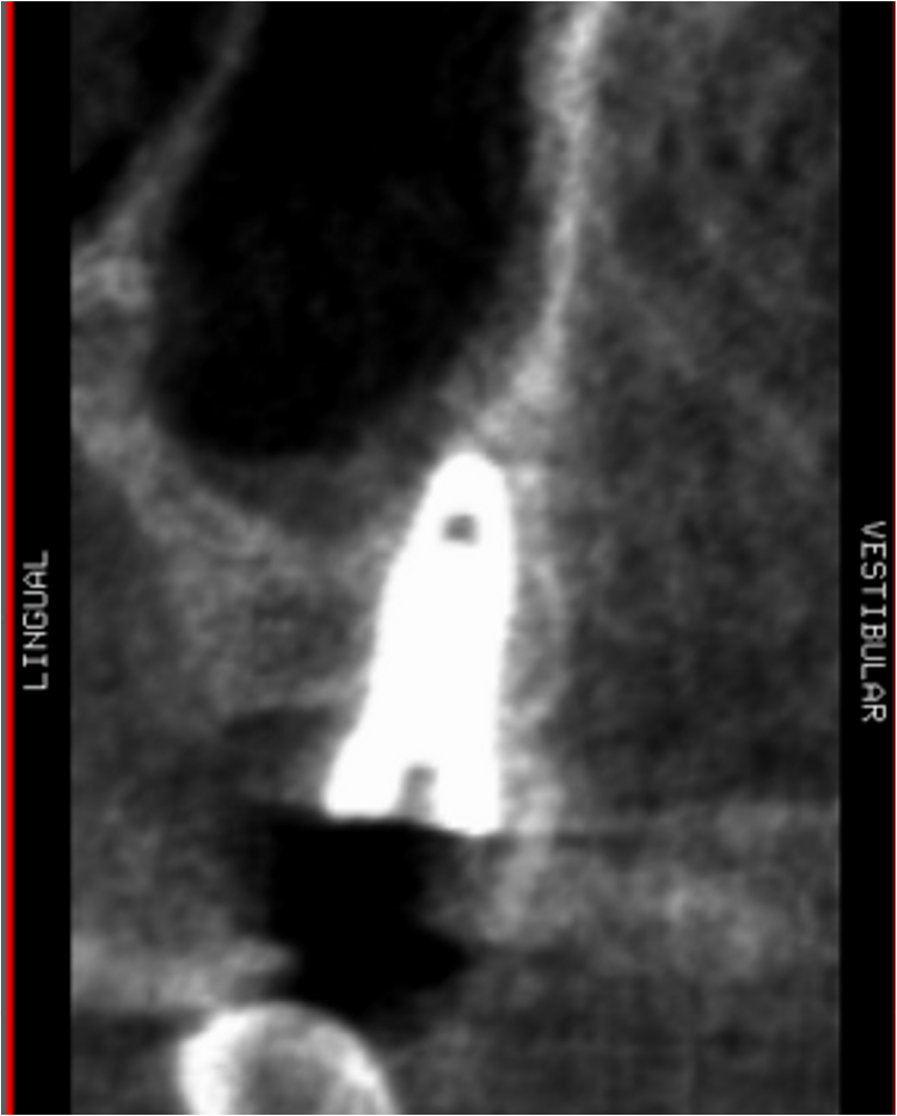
Postoperative implant position on CBCT
